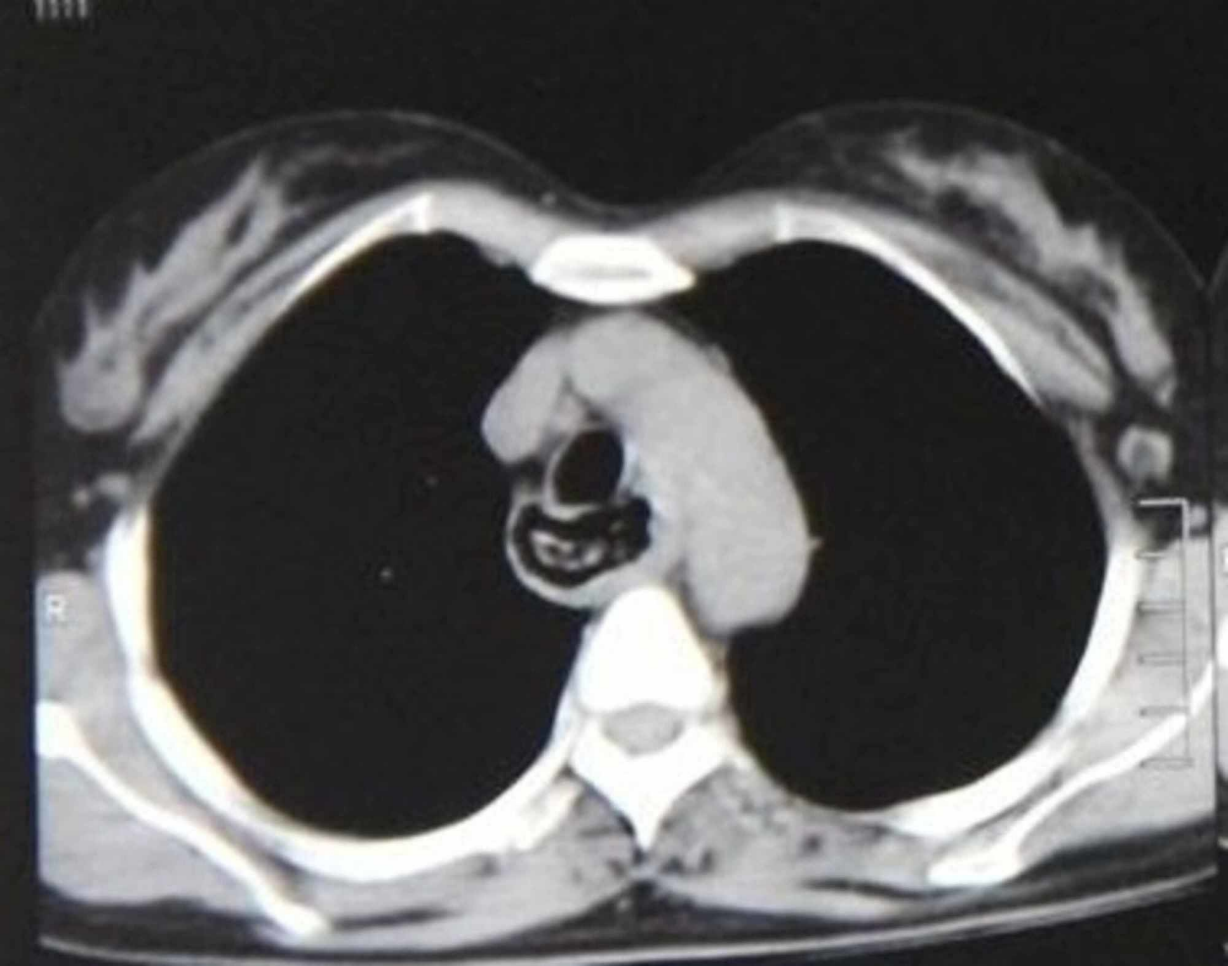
CT scan showing crescent-shaped foreign body in the esophagus
